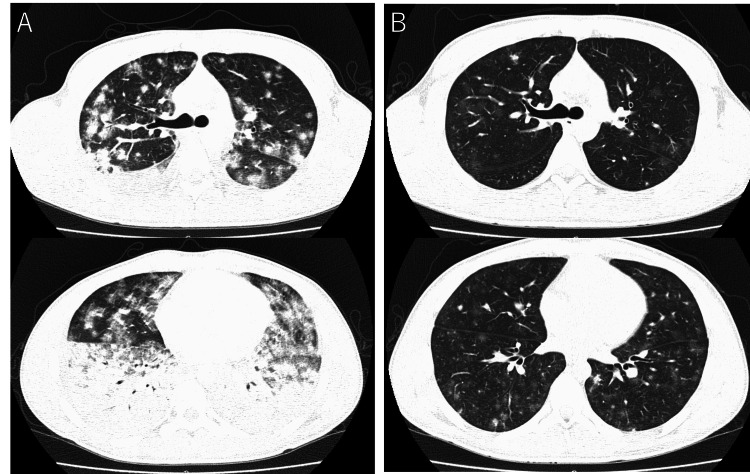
Chest CT A: On admission, chest CT showing progression of the diffuse ground-glass opacities and consolidation in both lower lung lobes. Some nodular shadows are also mixed. CT showed no hilar or mediastinal lymph node enlargement and a small amount of right pleural effusion. B: Hospital day 11, diffuse ground-glass opacities and consolidation in both lower lung lobes improved markedly.
